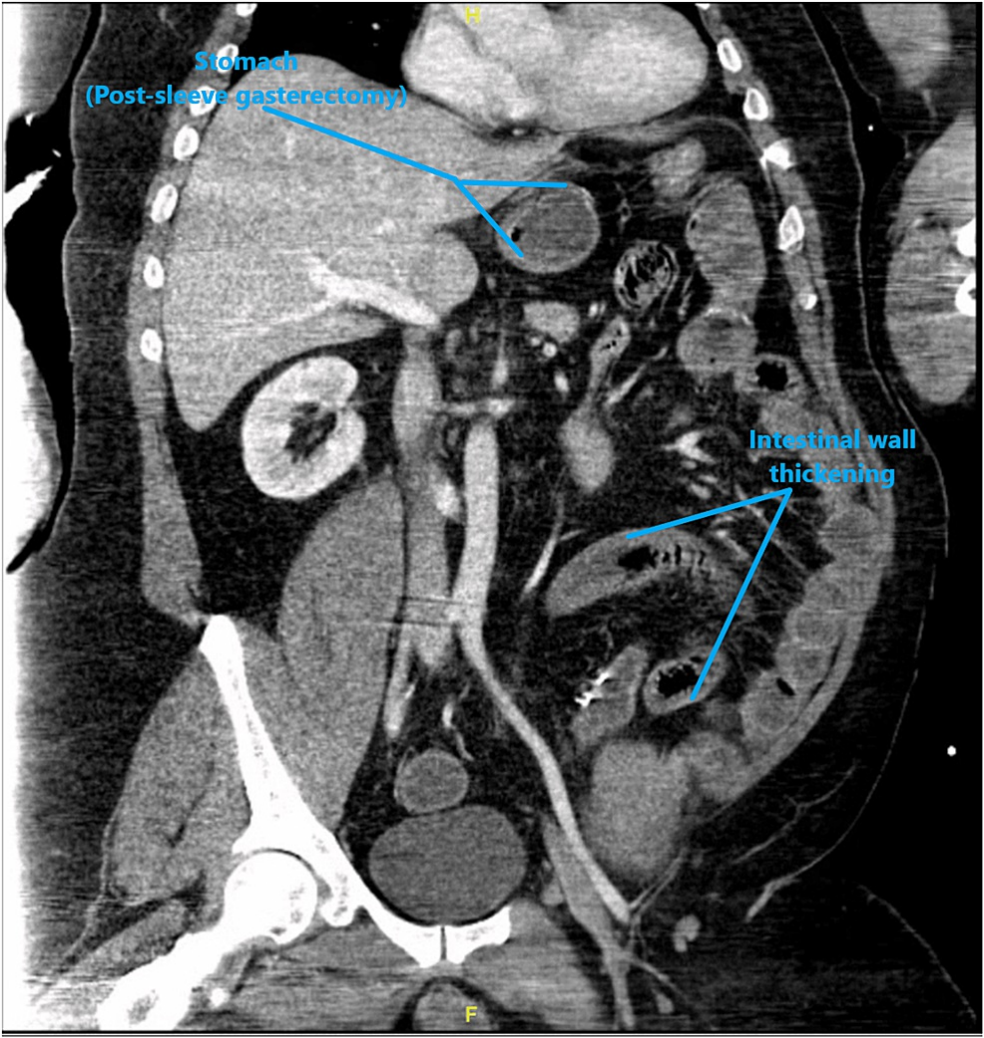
CT of the abdomen and pelvis with IV contrast with wall thickening of the small bowel (coronal section) IV: intravenous
